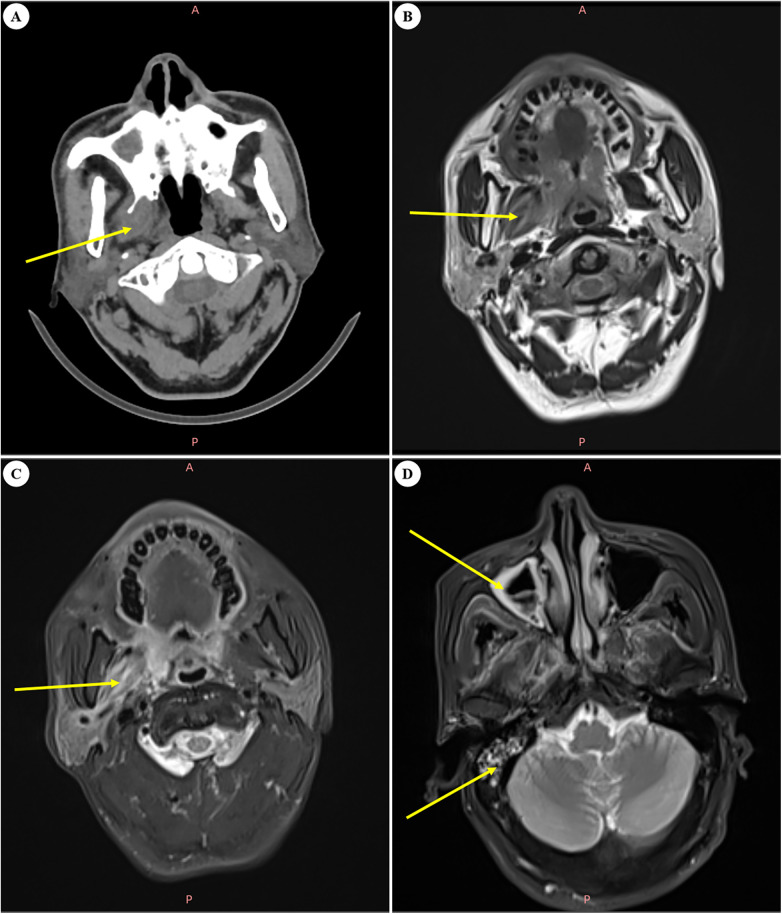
Imaging findings during the first hospitalization. (A) The right pterygoid muscle demonstrates increased volume with mild haziness in the adjacent adipose tissue (CT). (B) Edematous changes are observed in the right medial pterygoid muscle, exhibiting moderately elevated T2-weighted signal intensity in both the muscle and adjacent pharyngeal region (MRI). (C) Fluid-sensitive sequences reveal hyperintense signals localized to the right medial pterygoid muscle and pharyngeal space (MRI). (D) Concurrent inflammatory changes are noted, including right mastoiditis and right maxillary sinusitis (MRI).
